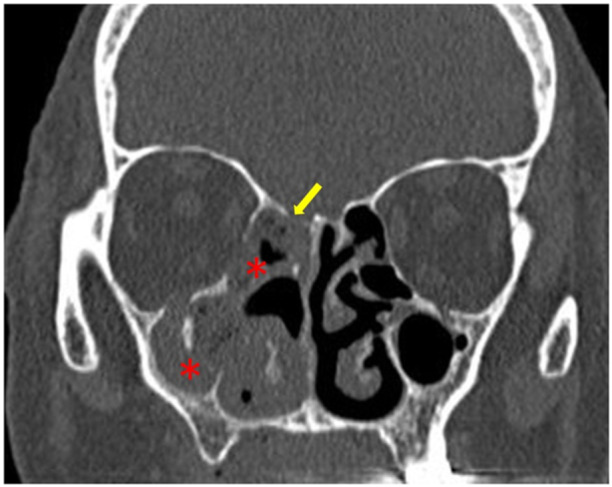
Coronal noncontrast CT image displayed in soft tissue and bone windows showing opacification of the right‐sided paranasal sinuses (*) with erosion of the cribriform plate (arrow).
